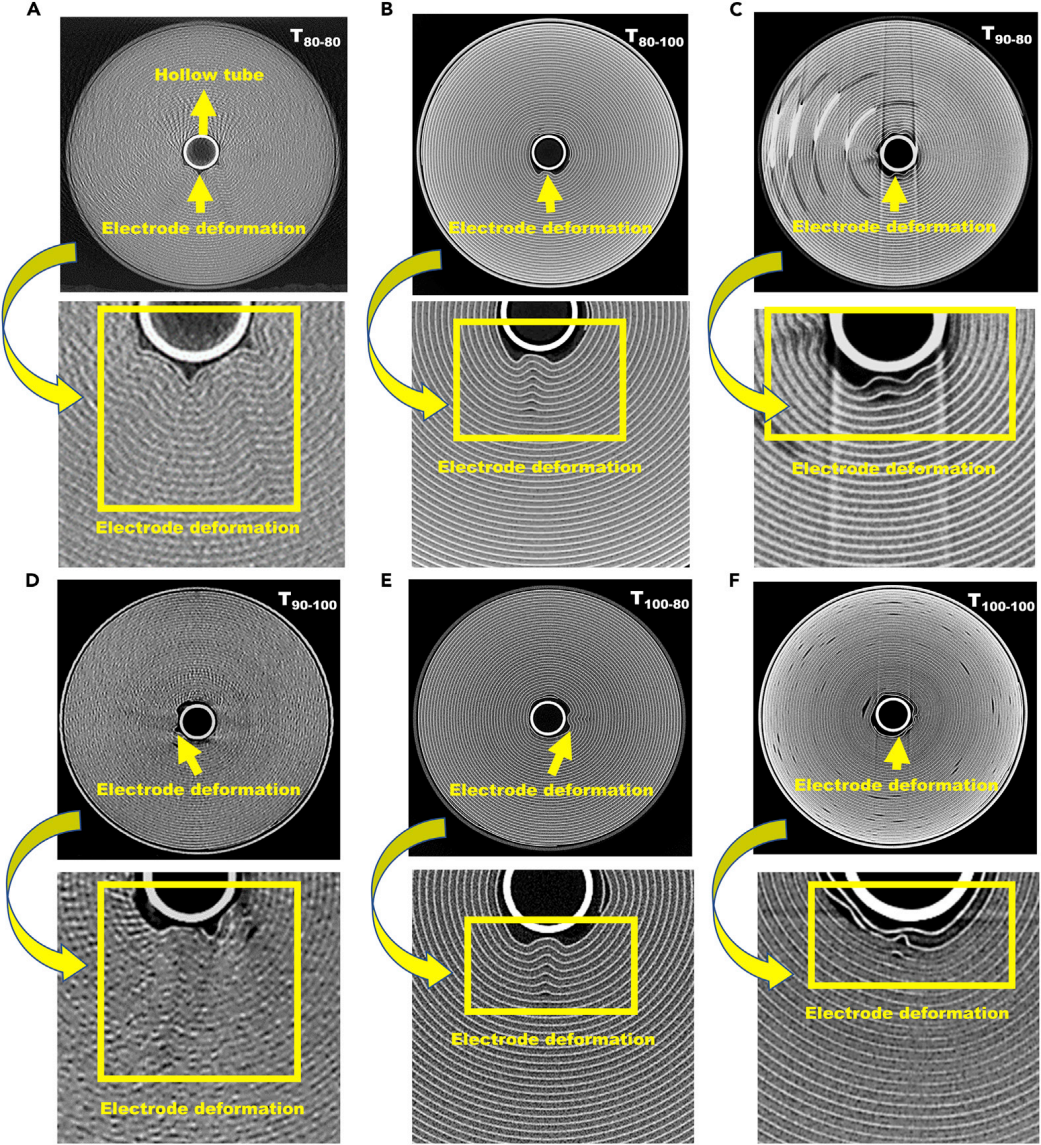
Cross-sectional CT images of the cell cycled at (A) T_80-80_, (B) T_80-100_, (C) T_90-80_, (D) T_90-100_, (E) T_100-80_, and (F) T_100-100_
